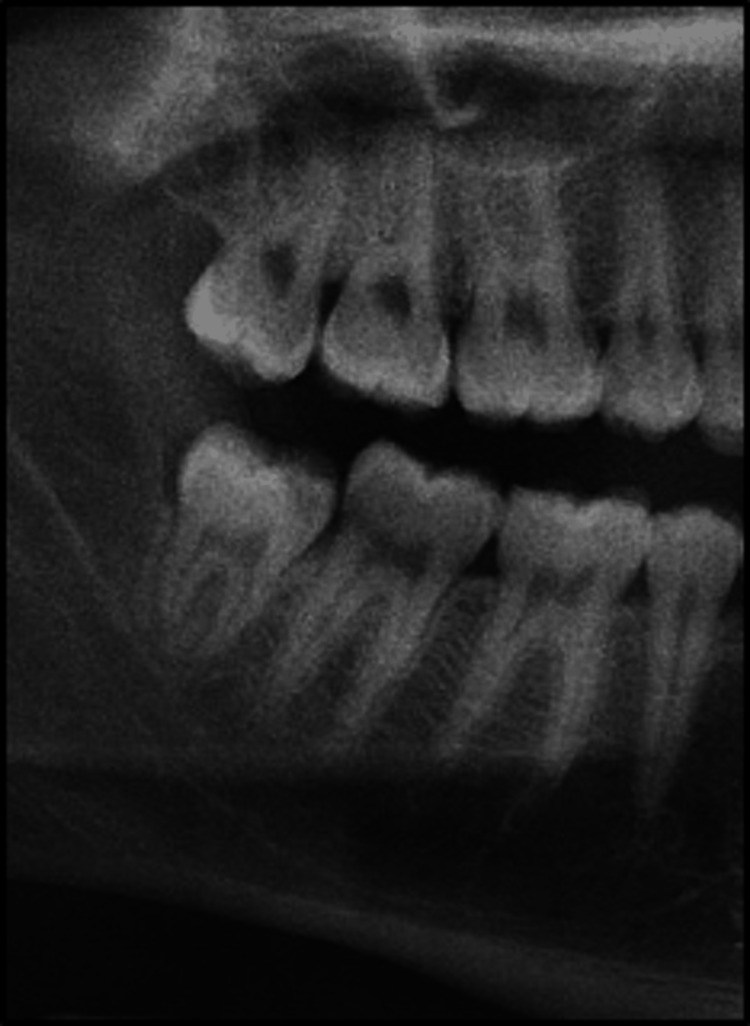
Preoperative cropped orthopantomogram
